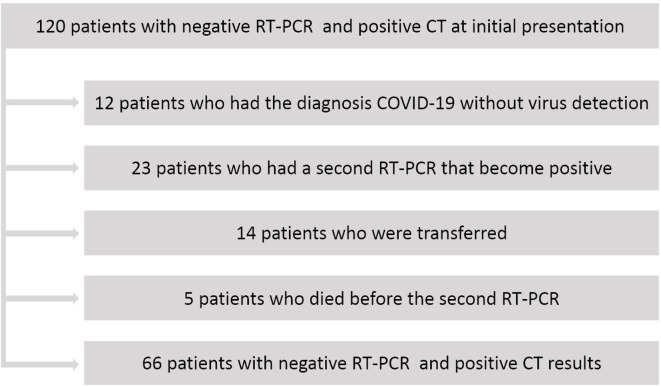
Subanalysis of patients with negative RT-PCR and positive CT at initial presentation (false-positive patients).
